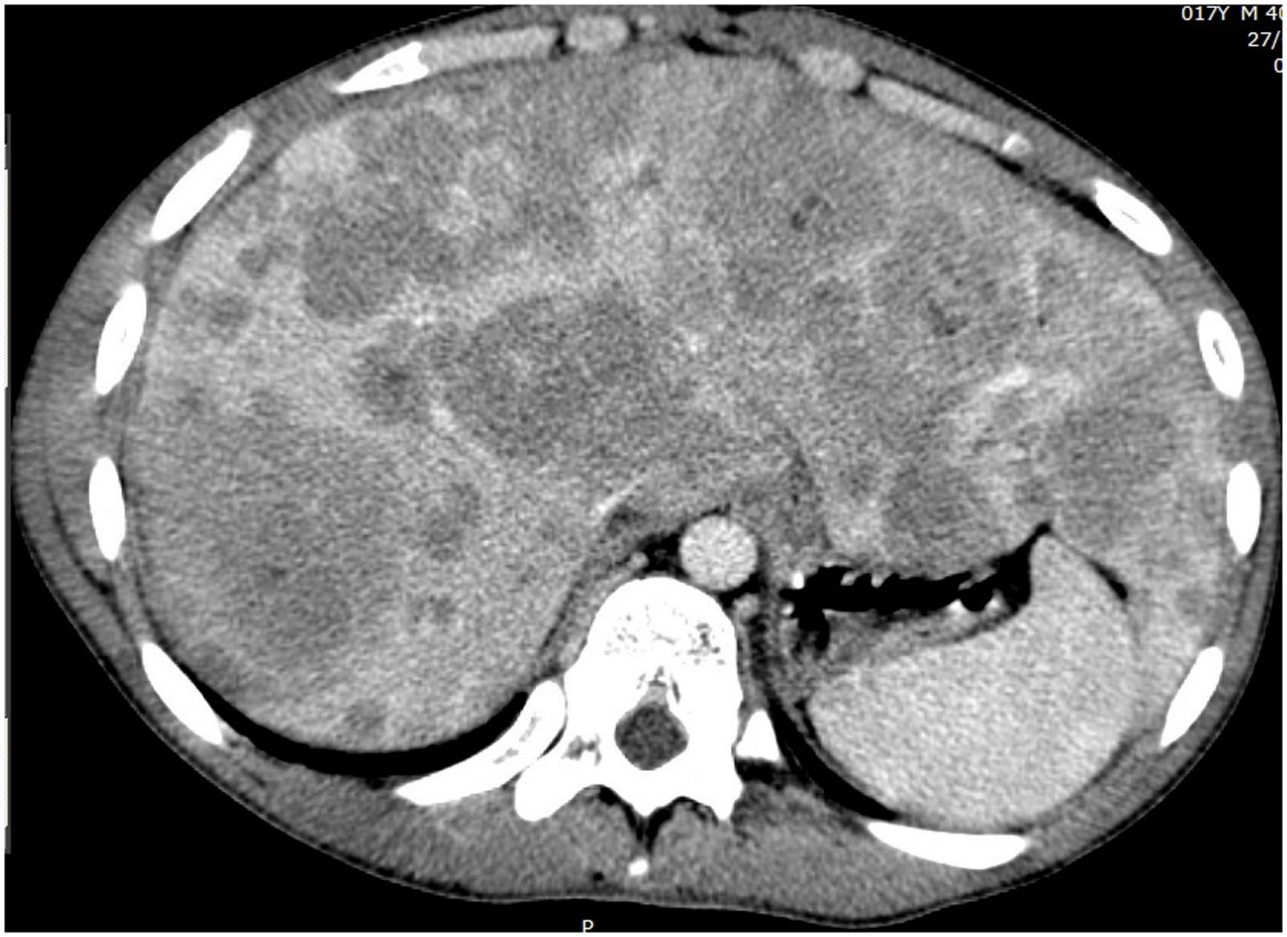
Abdominal CT with the multiple liver metastasis.
